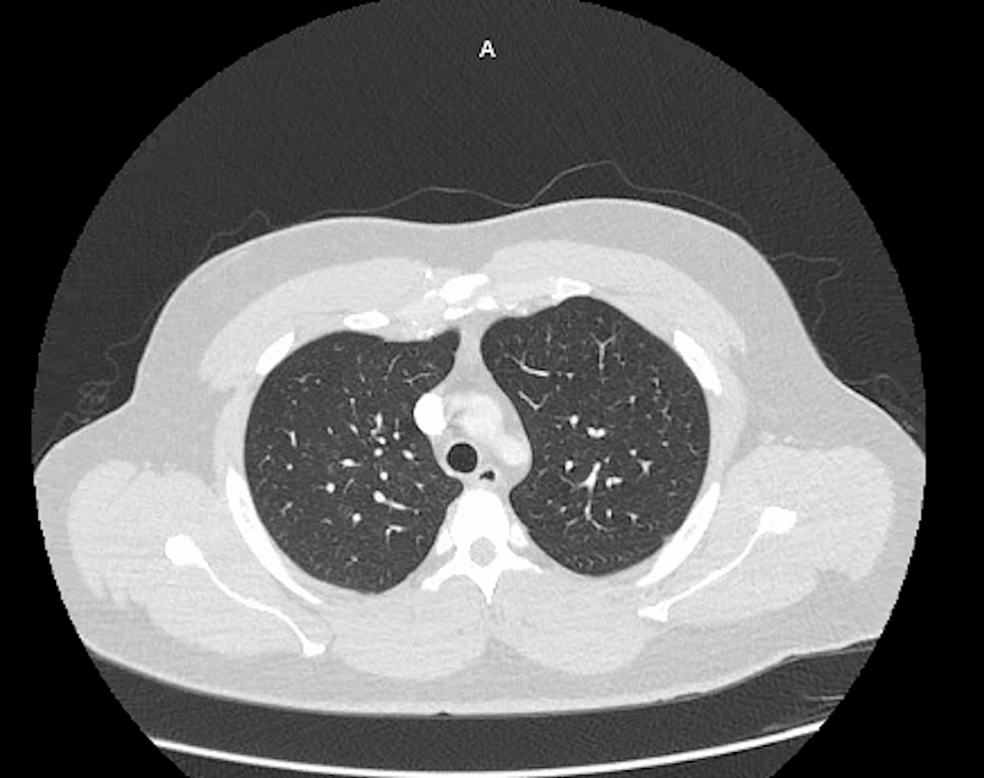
Chest computed tomography (CT) two weeks later, showing resolution of lesions of the right lung
